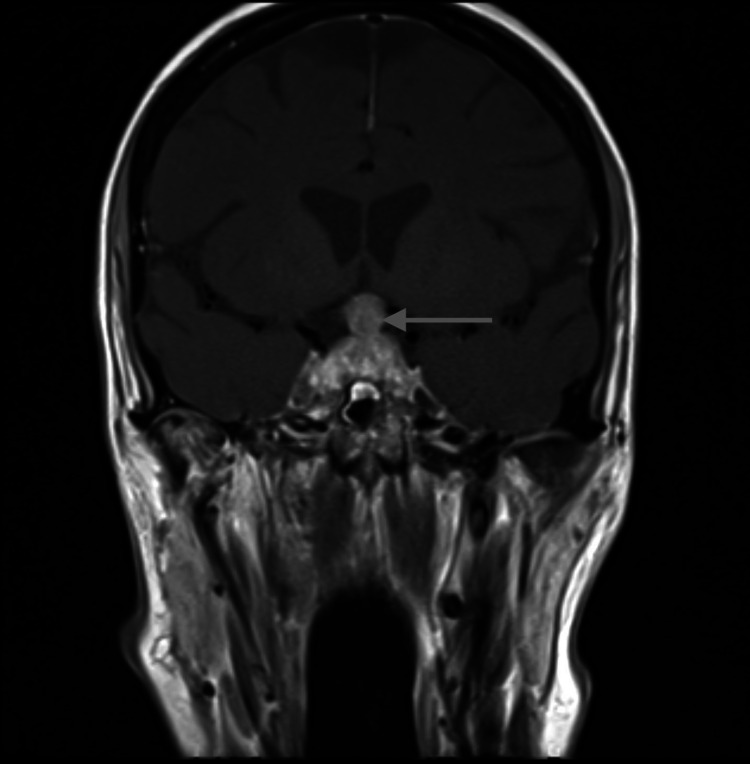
T1 post contrast brain MRI with pituitary protocol (coronal image) Image showing the mass effect on the optic chiasm and optic nerves Gray arrow: pituitary mass
